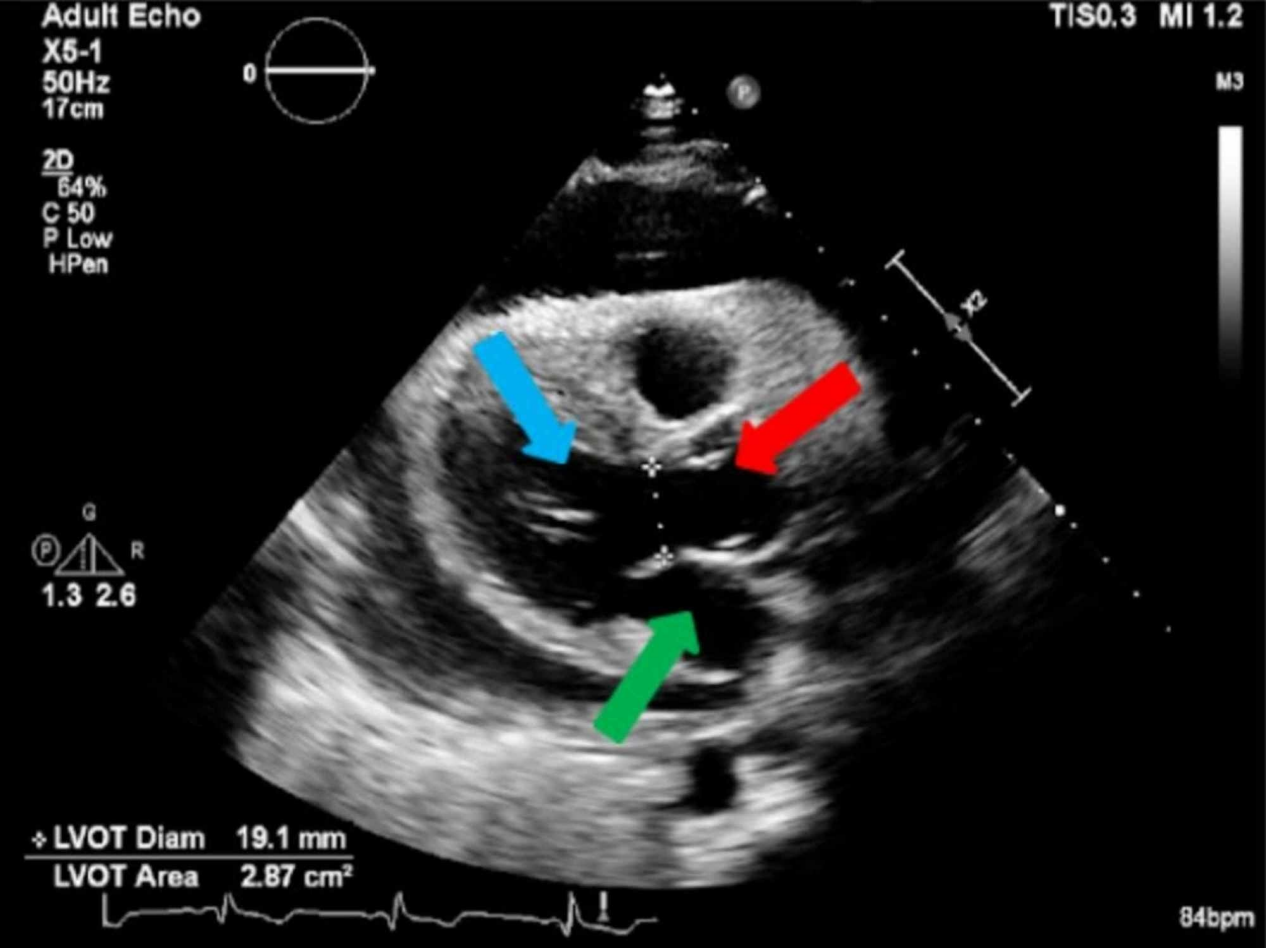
Parasternal long axis view of the left ventricle (blue arrow), aorta (red arrow), and the left atrium (green arrow).
